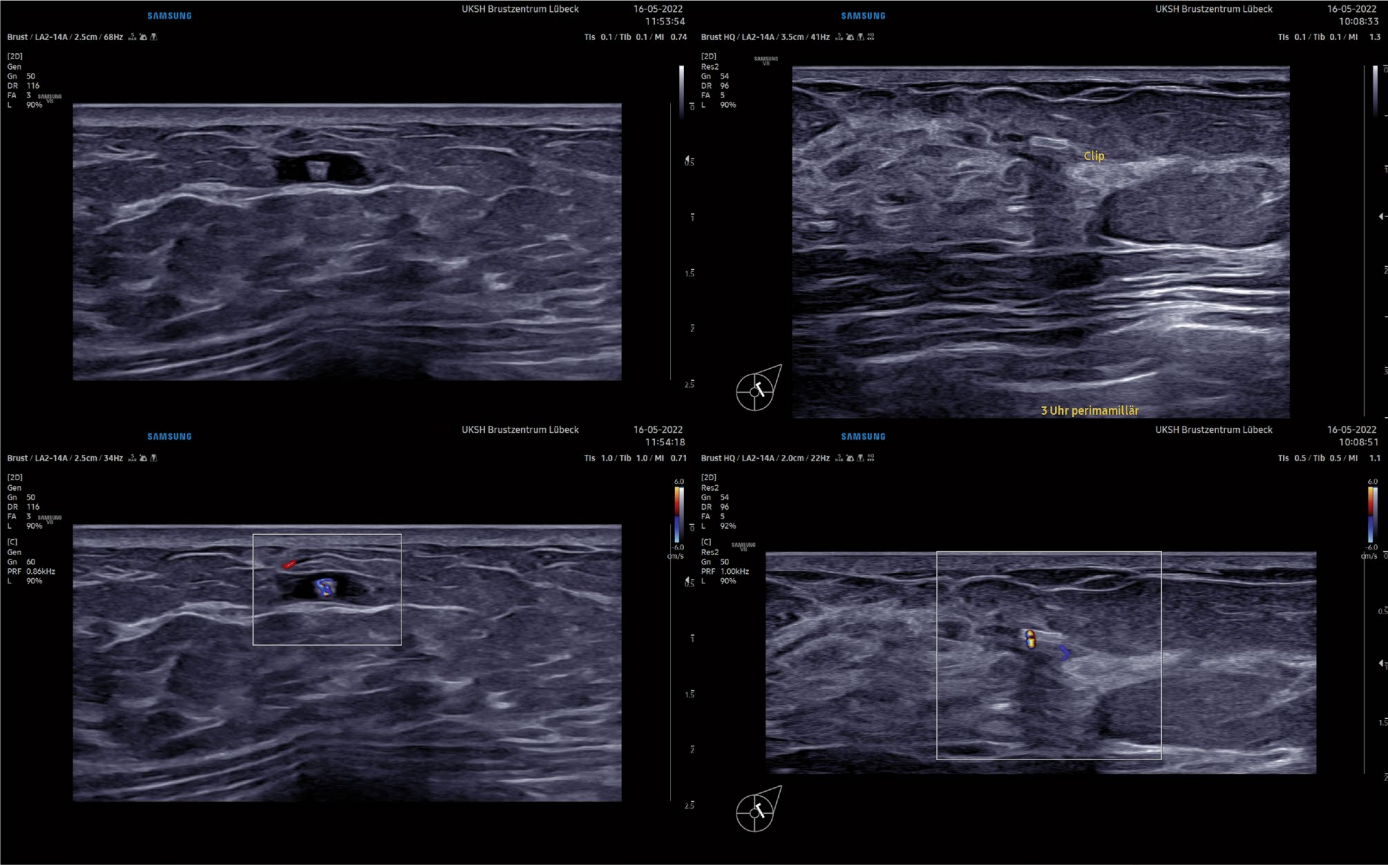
Two different clips shown in B-mode (upper row) and using color Doppler (lower row). Left: small cavernous hemangioma, confirmed by core-biopsy with synchronous clip placement. The visible lesion with the clip was localized intraoperatively via ultrasound without the necessity for a preoperative localization step with, e.g., a wire. Right: core-biopsy confirmed breast cancer with complete remission upon imaging. The clip was placed before start of neoadjuvant chemotherapy
